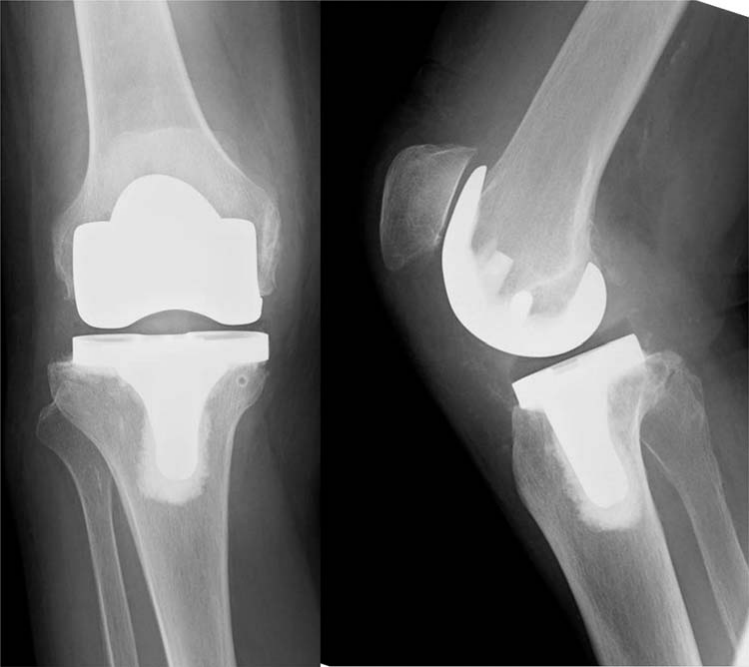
X-ray examination showed a patellar alta.
